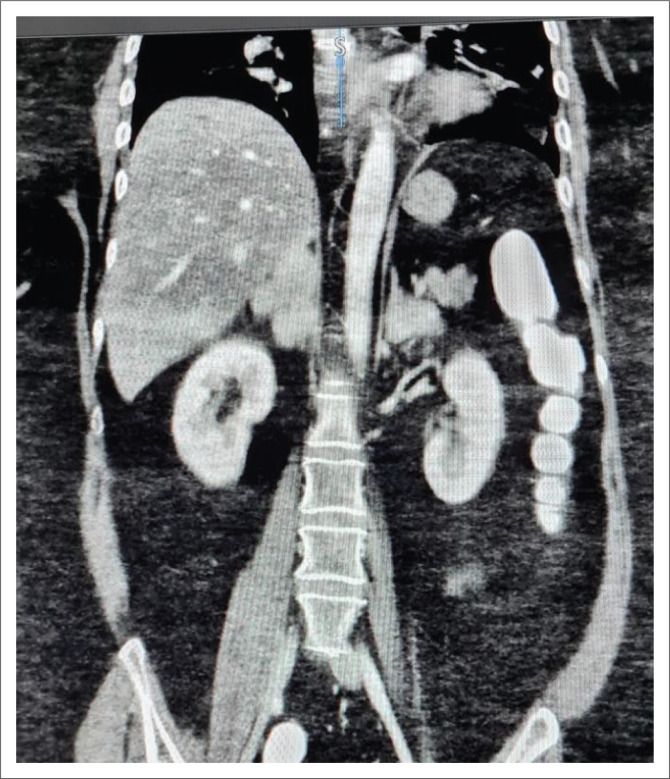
Computed tomography (coronal view) demonstrating bilateral adrenal hyperplasia with the right adrenal gland measuring 44.7 mm × 30.3 mm and the left adrenal gland measuring 25.0 mm × 27.7.mm.
